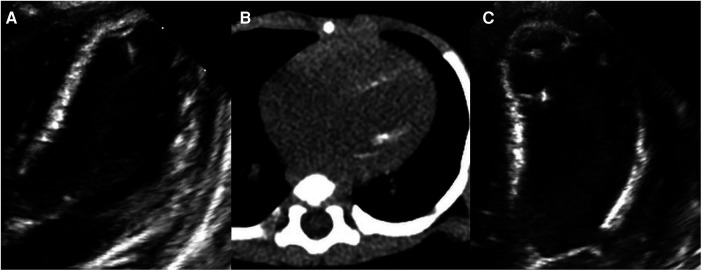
Left ventricular (LV) calcification detected by echocardiography and computed tomography angiography (CTA). At 2.5 months of age, (A) echocardiography showing increased echo of LV endocardium and (B) CTA showing diffusely distributed calcification of the LV endocardium. At 4 months of age, (C) echocardiography showing diffusely distributed calcification of the LV endocardium, chordae tendineae, and papillary muscle.
